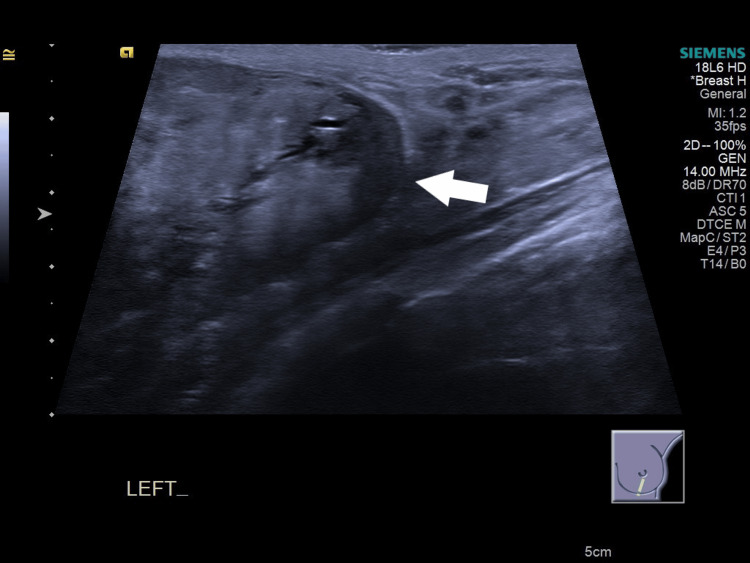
A high-resolution ultrasound image showing part of the lower margin of the left breast mass. The lesion is heterogeneous and predominantly solid with a few small cystic areas. The margins of the mass are smooth (arrow).
